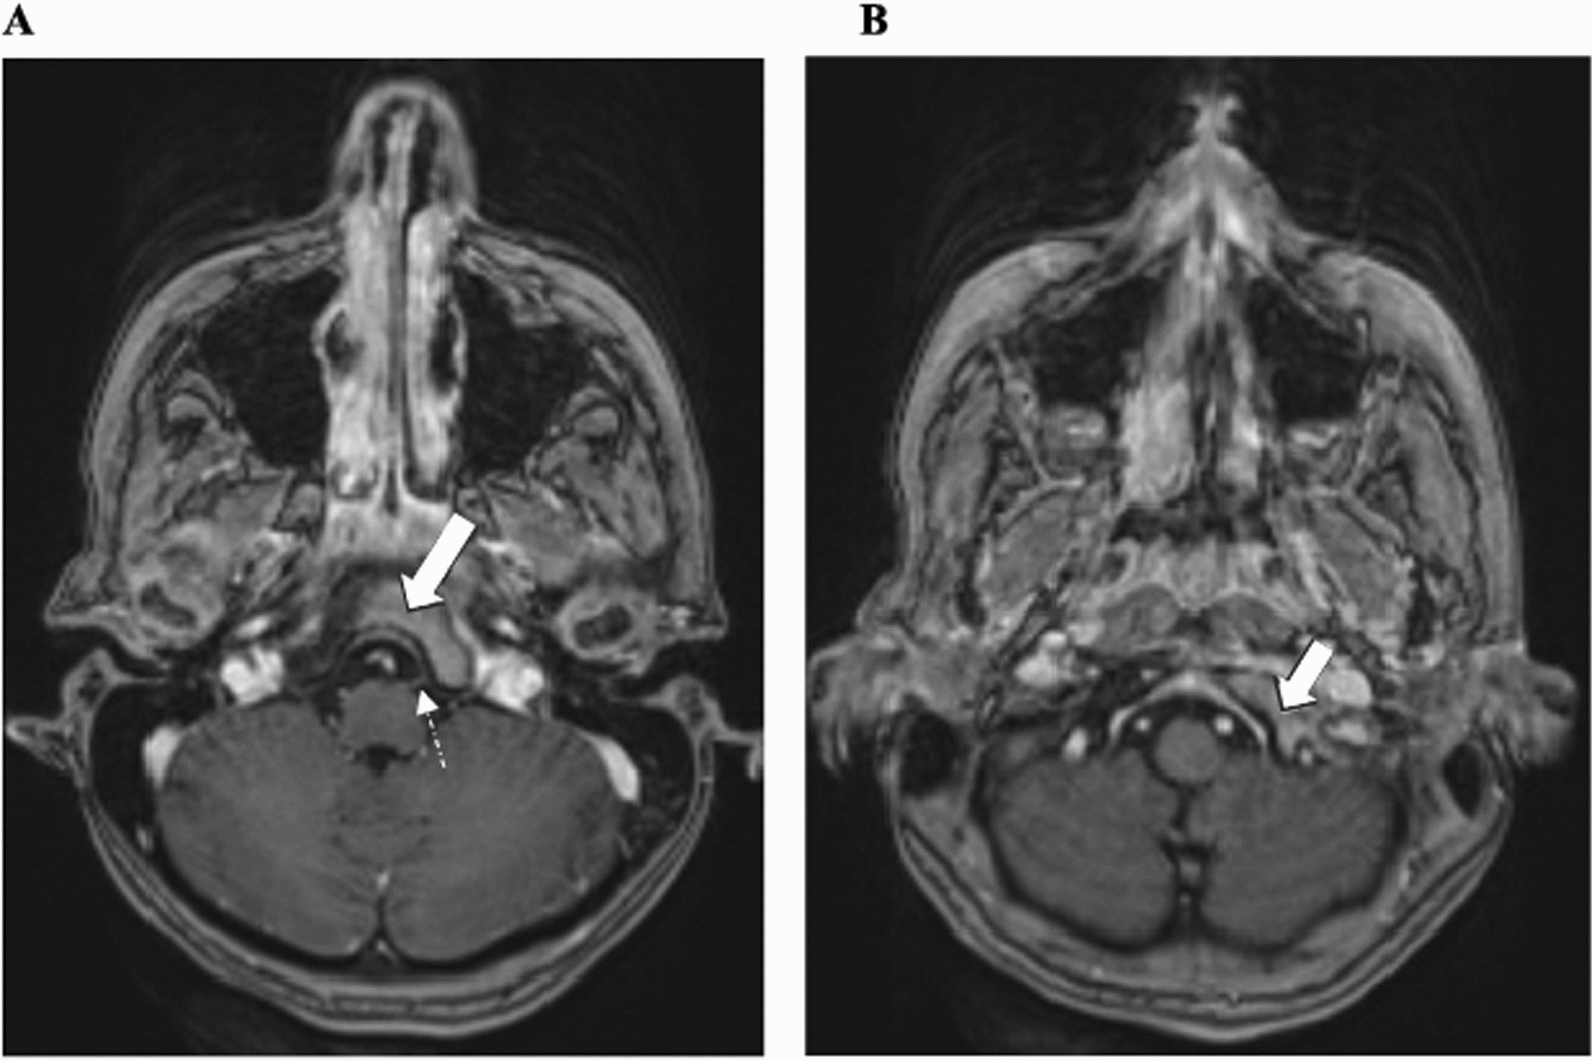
Magnetic resonance imaging with fast imaging employing a steady-state acquisition sequencing indicating the involvement of an aggressive infiltrative osseous lesion on the clivus of the left occipital bone near the hypoglossal canal. (A) Asymmetric left clivus bone enhancement. The left hypoglossal nerve can be visualized and is non-enhancing (exiting the ventral medulla, marked by a dotted arrow), (B) demonstrating asymmetric left occipital bone enhancement compared with the right
